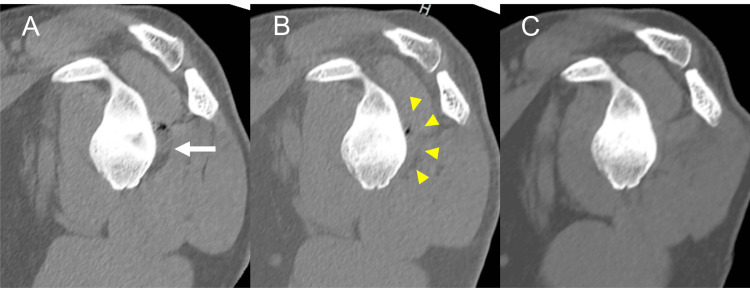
Radiological course of the cystic lesion protruding into the spinoglenoid notch The cystic lesion (arrow) on the preoperative CT (A) was resected, but a small space occupied by a suspected hematoma remained (triangle heads) by operation (B). (C) No lesion recurrence was reported at the six-month follow-up.
